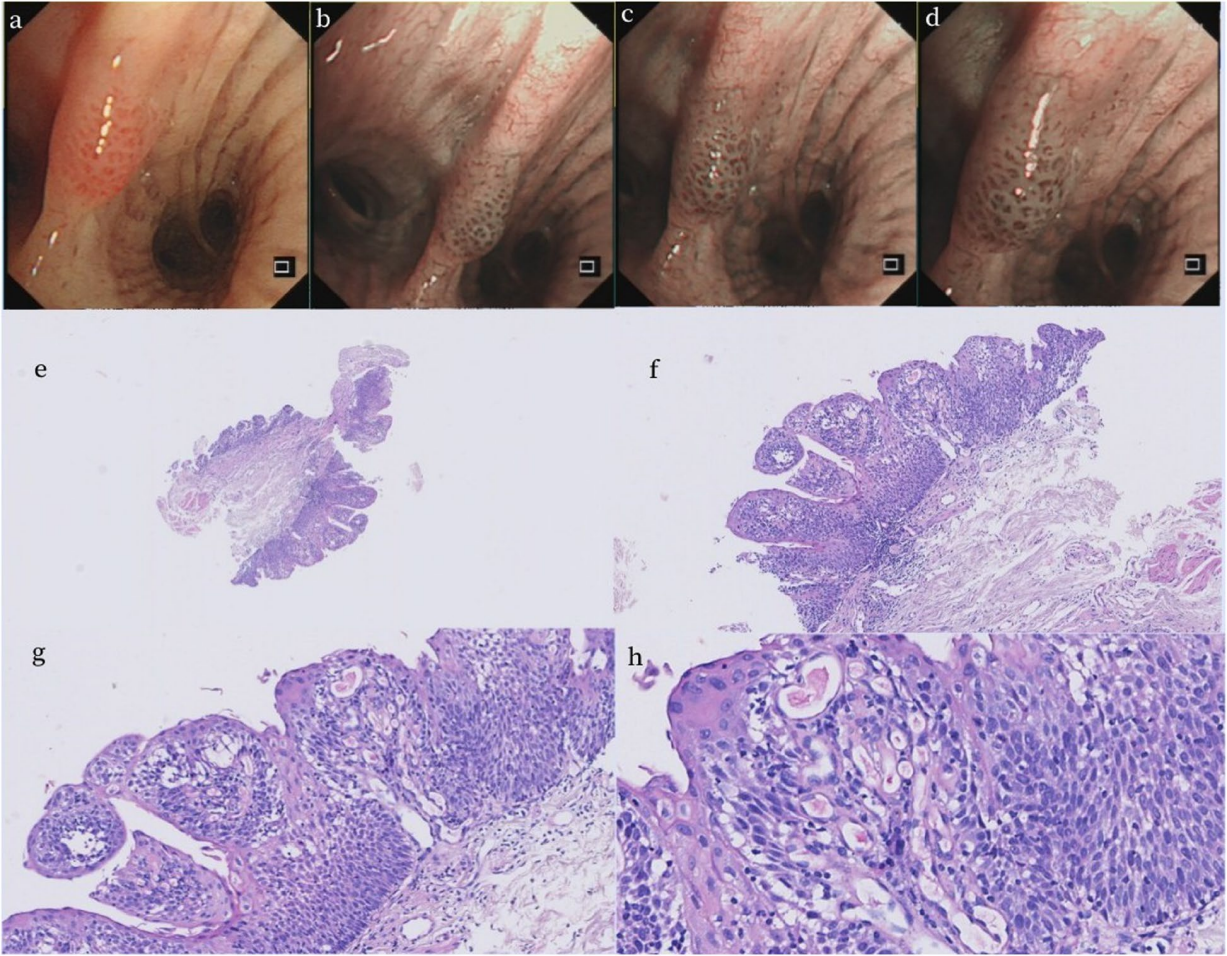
Bronchoscopic and pathological images of a CT-occult lesion. The patient was a 65-year-old male with a history of heavy smoking for more than 40 years. He was admitted to the hospital due to pulmonary nodules found in physical examination, and CT occult bronchial mucosal lesions of the central airway were found during bronchoscopy. a Conventional white light mucosal lesion morphology under bronchoscopy. b-d Mucosal vascular morphology under narrow-band imaging mode under bronchoscopy. e–h Pathological section images magnified 40 times, 100 times, 200 times and 400 times, respectively, Pathological diagnosis was mucosal squamous epithelial papillary hyperplasia with local mild dysplasia
